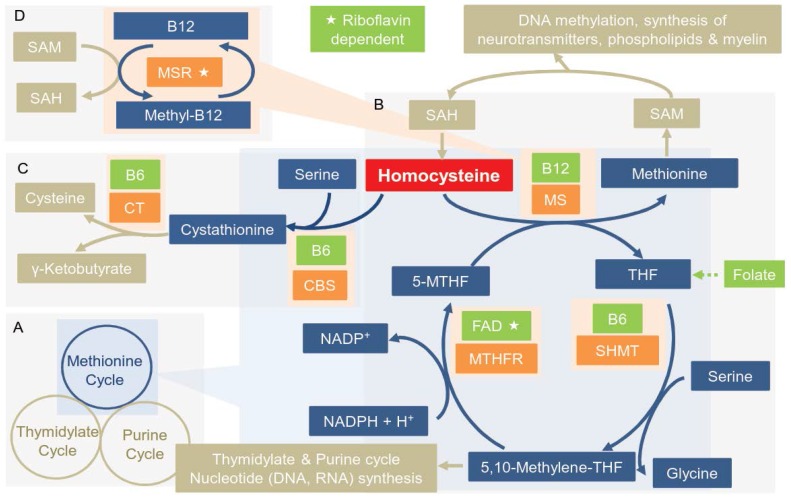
Metabolic pathways of the one-carbon metabolism: (A) Overview of the three cycles; (B) Methionine cycle: remethylation of homocysteine to methionine; (C) Transsulfuartion pathway: Irreversible conversion of homocysteine into cysteine; (D) Remethylation of vitamin B12 to its active form; CBS: cystathionine β-synthase; CT: γ-cystathionase; FAD: flavin adenine dinucleotide; MTHF: methylenetetrahydrofolate; MTHFR: methylenetetrahydrofolate reductase; MS: methionine synthase; MSR: methionine synthase reductase; NADP(H): (Hydroxy) Nicotinamide adenine dinucleotide phosphate; SAH: *S*-adenosylhomocysteine; SAM: *S*-adenosylmethionine; SHMT: serine hydroxymethyltransferase THF: tetrahydrofolate.
